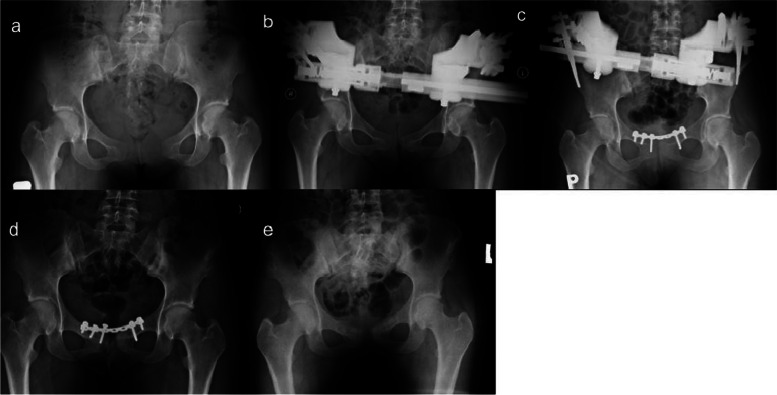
Case 2. A 25-year-old woman was admitted to the department 3 months after delivery. a Initial plain radiograph showing the pubic symphysis diastasis (APC II). b A radiograph was taken after performing closed reduction using an external fixator, resulting in a symphysis diastasis of 12 mm. c An additional internal fixation was performed using a 6-hole plate to reduce remaining diastasis. d Eleven-month follow-up radiograph shows a 12 mm gap in the reduction of the pubic symphysis. e One year after the removal of screws and a plate
